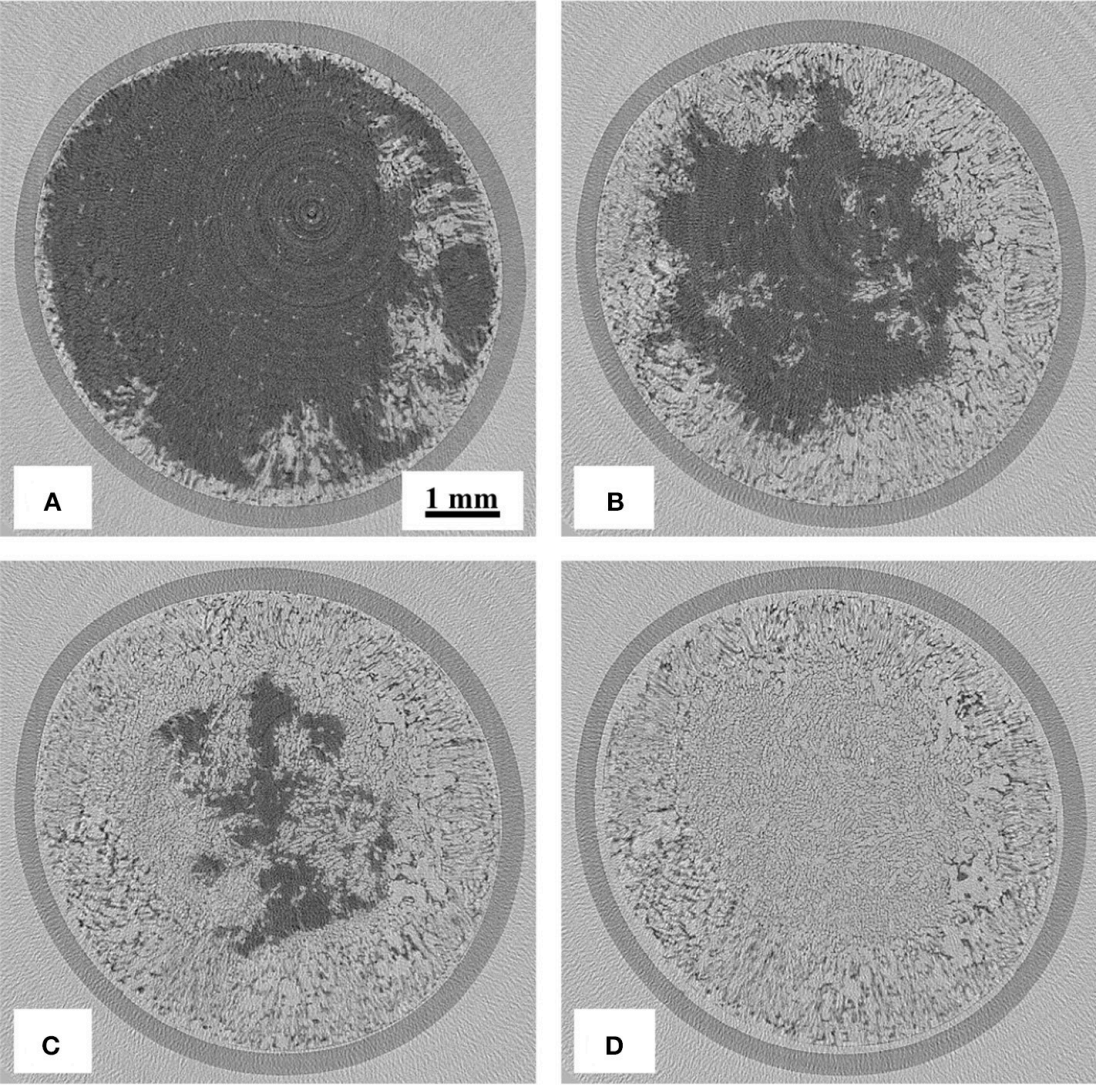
2D X-ray CT images during freeze-drying of the samples annealed for 12 h (horizontal cross-section at 1,500 μm from the top), dried for (A) 1,800, (B) 2,400, (C) 3,000, and (D) 3,900 s.
